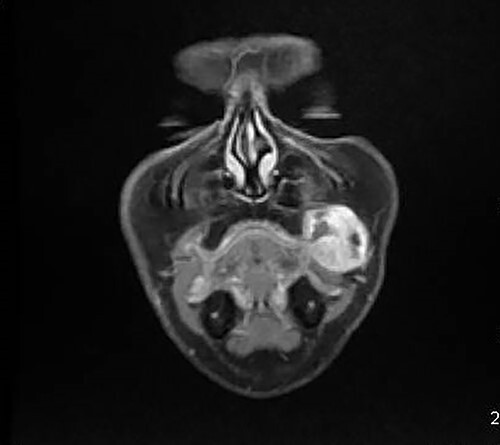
MRI showing the tumor of the left cheek with contrast enhanced.
